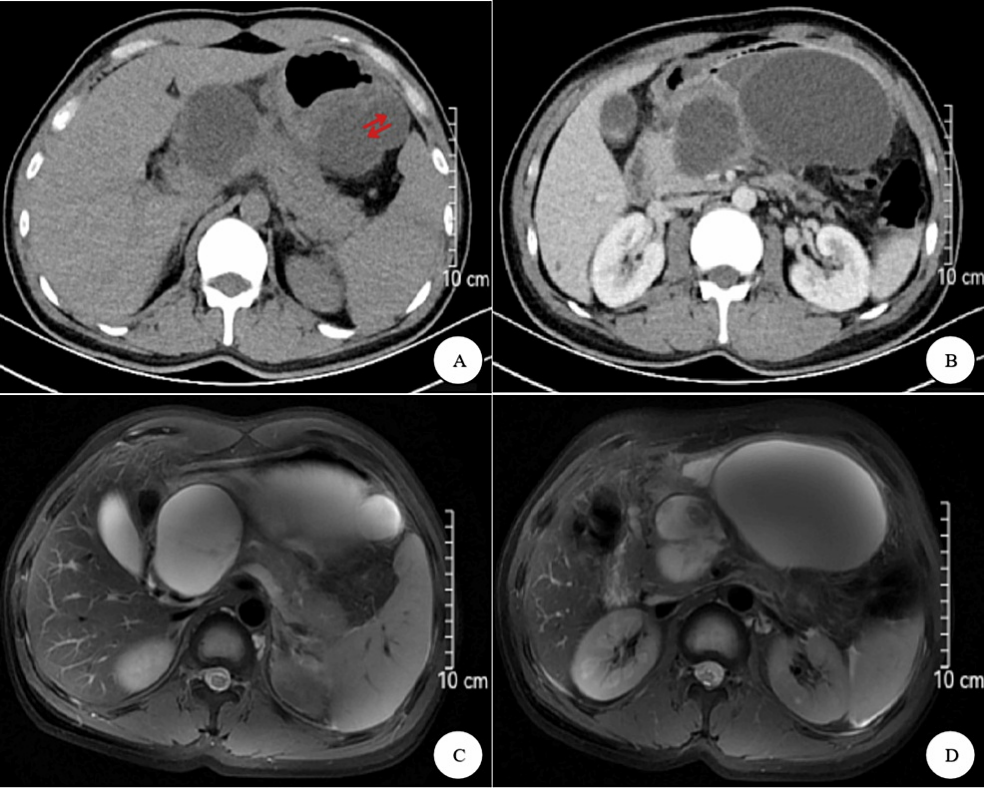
Abdominal CT and MRI, presented in coronal scans, revealed one giant and multiple small pancreatic pseudocysts distributed across the head, body, and tail of the pancreas. The largest pseudocyst, measuring approximately 122 x 79 mm, was situated in the body of the pancreas and communicated with the tail, as indicated by the red arrow.
